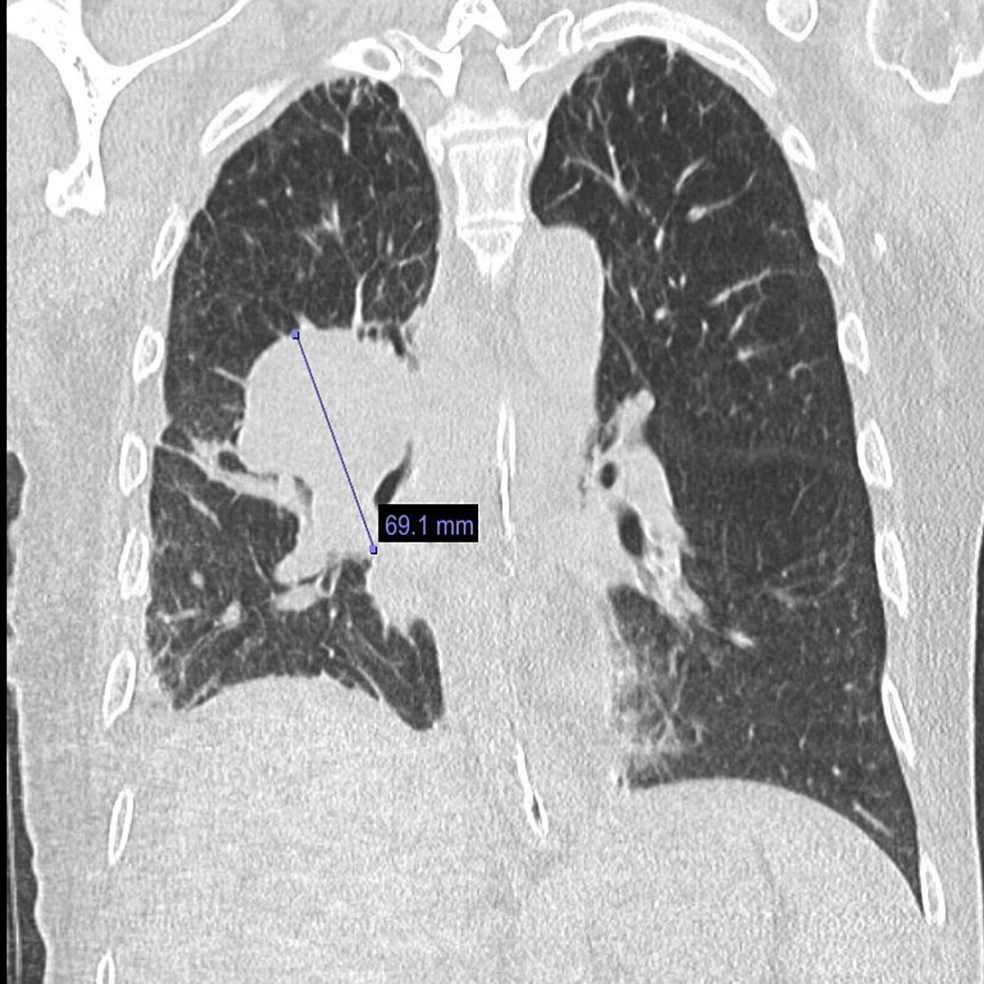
CT scan of the chest showing interval increase in the size of the right lung mass. CT: computed tomography
